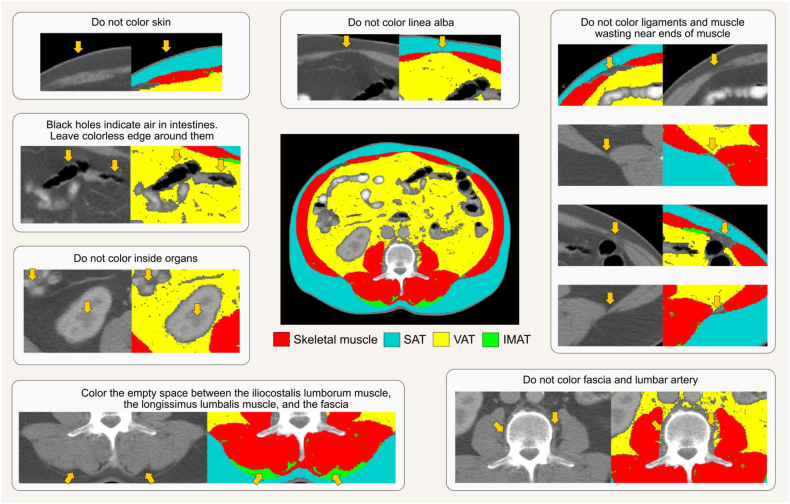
Important considerations for body composition assessment at the third lumbar vertebrae using a computerized tomography (CT) scan. IMAT, intermuscular adipose tissue; SAT, subcutaneous adipose tissue; VAT, visceral adipose tissue.
